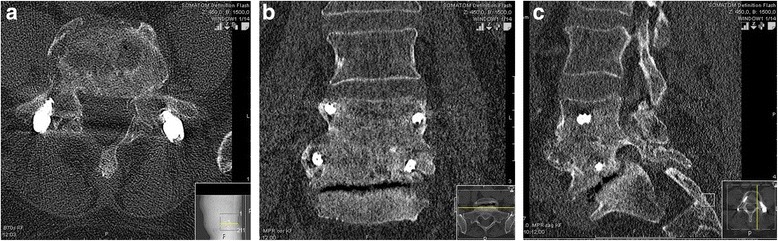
a, b and c Follow-up computed tomography scan of the lumbar spine at 11 months on 7 Dec 2015: consistent position of the posterior instrumentation. Complete bony fusion of L4 and L5
